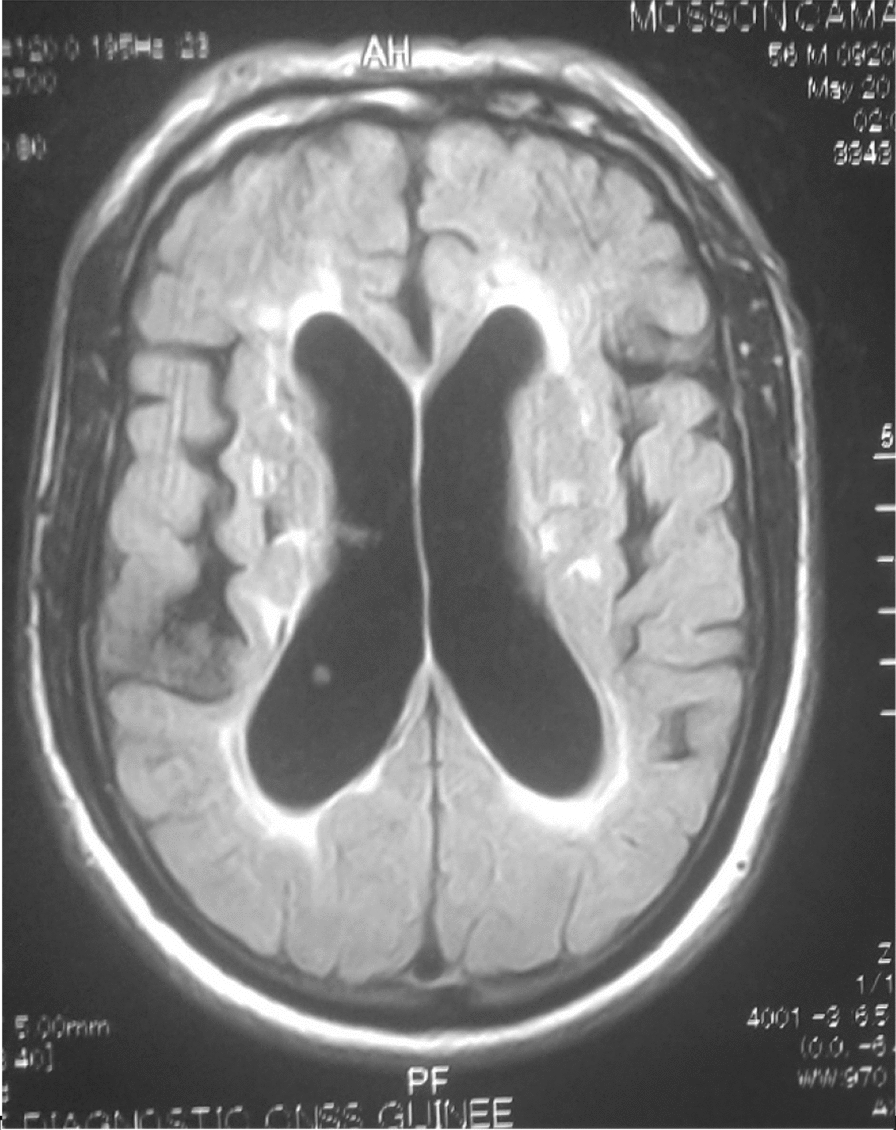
Cerebral MRI: periventricular hypersignals on the T1-weighted image
